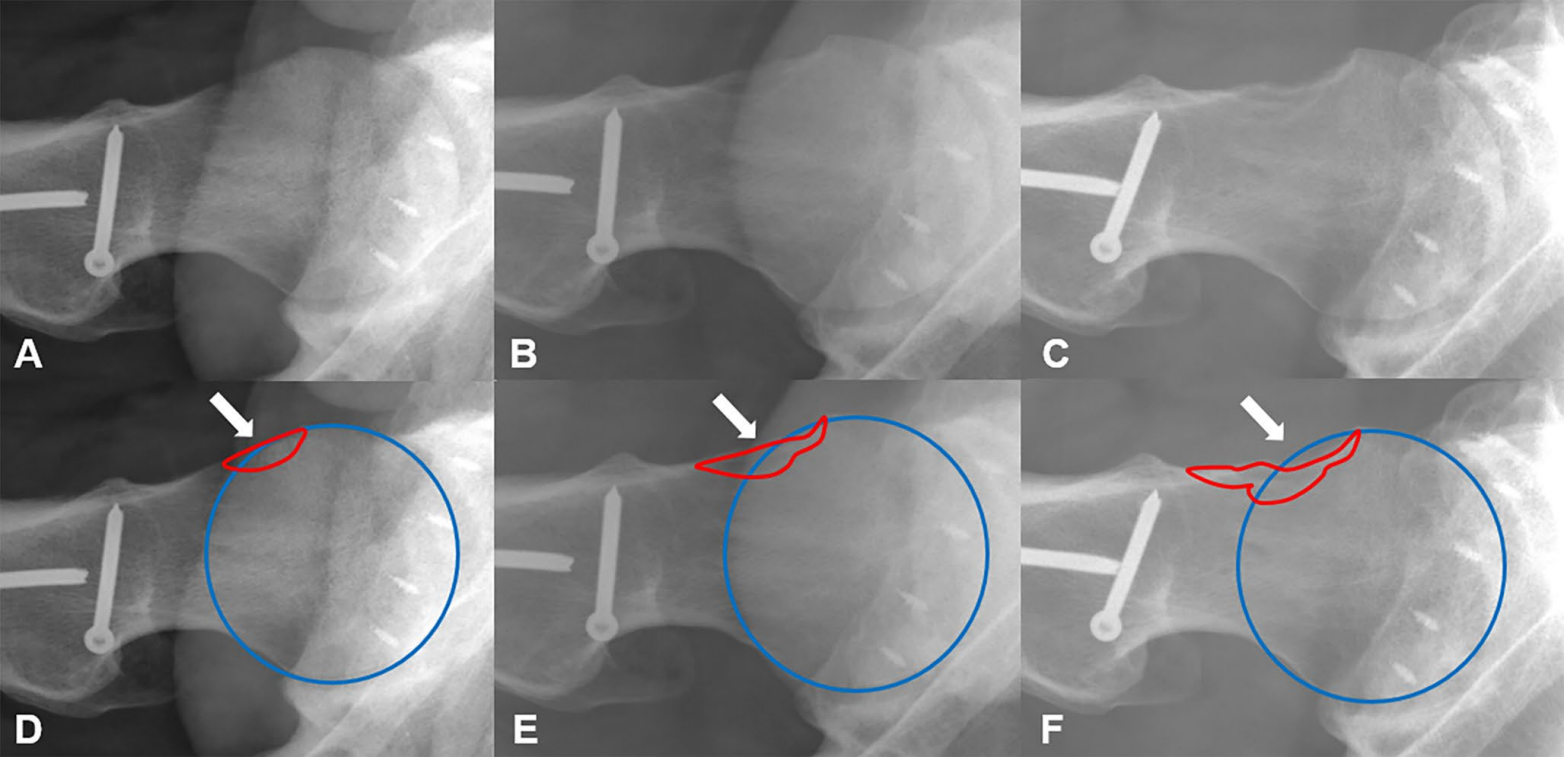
Comparison of area of femoral head and osteolysis over the years. A: Initial X-ray after offset-correction, B: X-ray five years after offset correction, C: X-ray 10 years after offset correction, D: X-ray after offset-correction with measurements of area of femoral head (blue) and initial area of offset correction (red), E: X-ray five years after offset correction with measurements of area of femoral head (blue) and osteolysis (red), F: X-ray 10 years after offset correction with measurements of area of femoral head (blue) and osteolysis (red)
